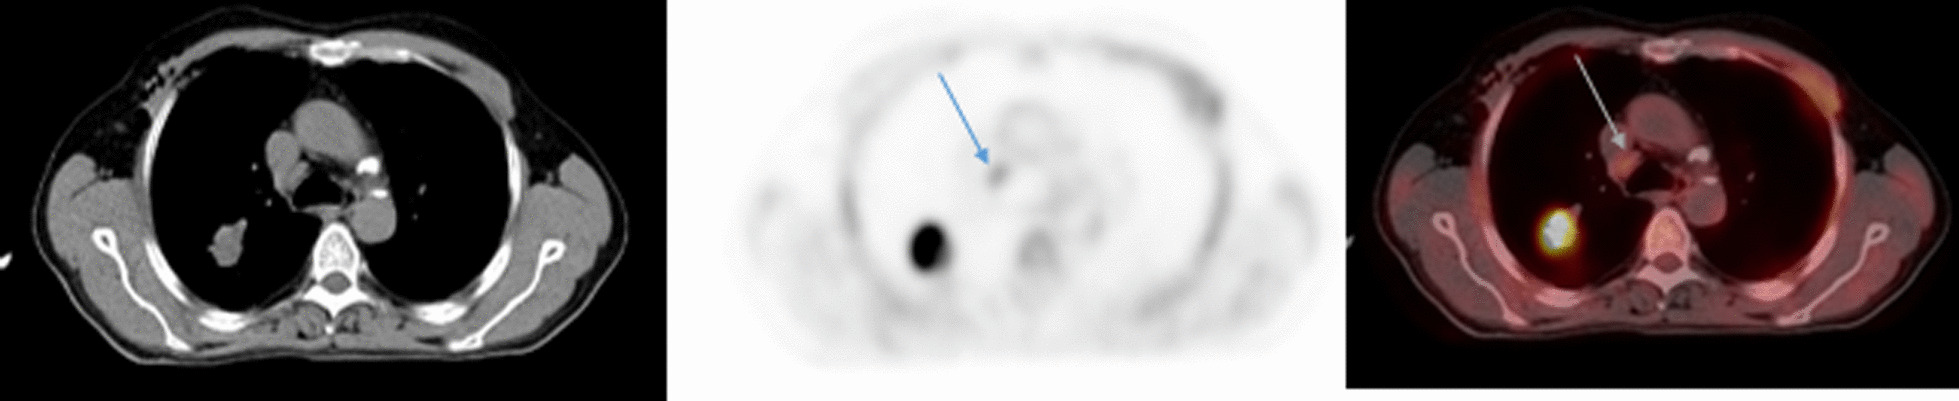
False positive FDG-PET/CT and true negative EBUS/TBNA. A case of right lung moderately differentiated lung adenocarcinoma. Axial CT scan showed prominent right lower paratracheal lymph node. Axial FDG-PET and FDG-PET/CT showed suspicious hypermetabolic (SUVmax: 3) right lower paratracheal lymph node. EBUS/TBNA turned negative for lymph node metastases. Lymph node dissection confirmed the EBUS/TBNA result
